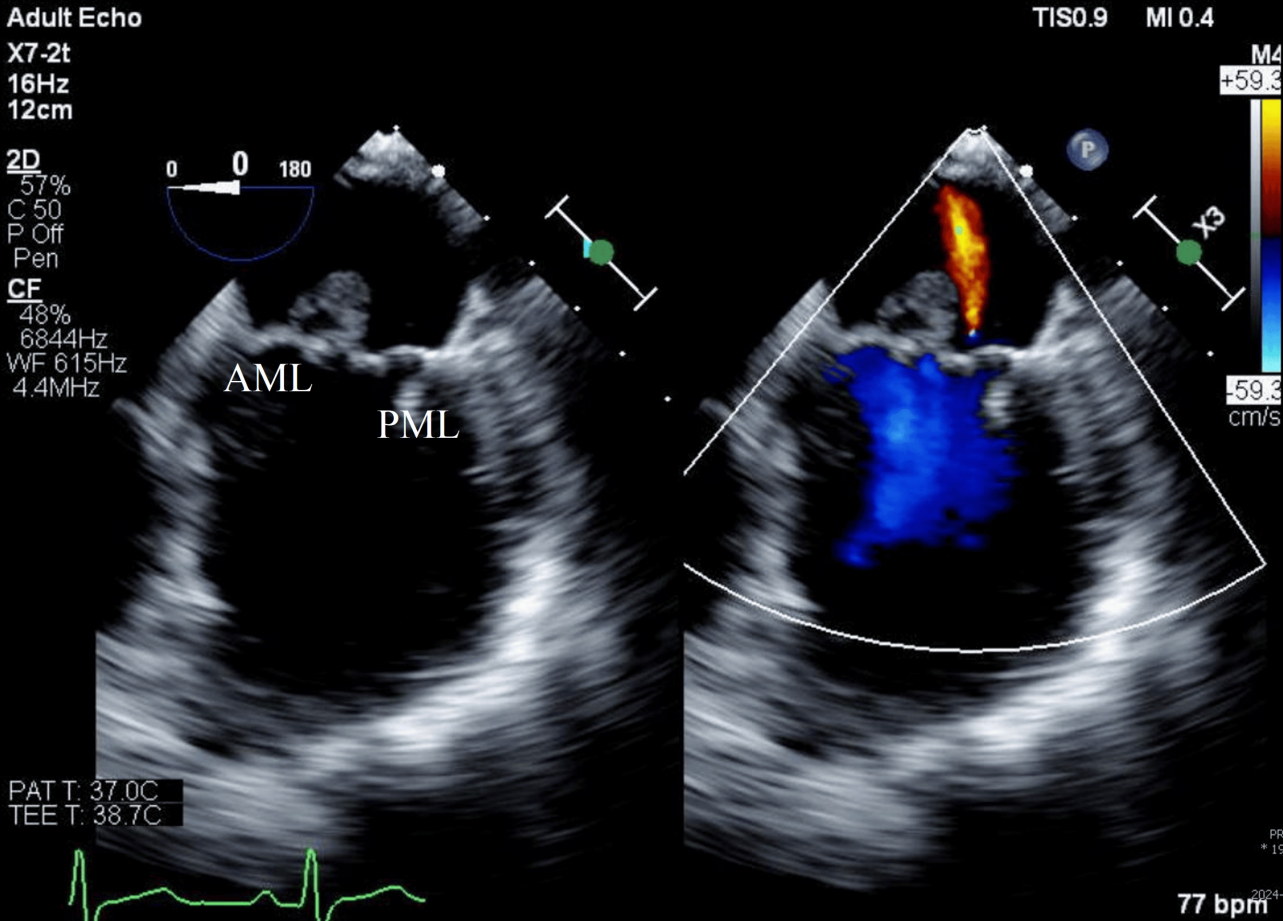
TEE mid-esophageal four-chamber view, mitral valve-focused showing large vegetation on the mitral valve with color Doppler showing mitral regurgitation TEE: transesophageal echocardiography, AML: anterior mitral leaflet, PML: posterior mitral leaflet
